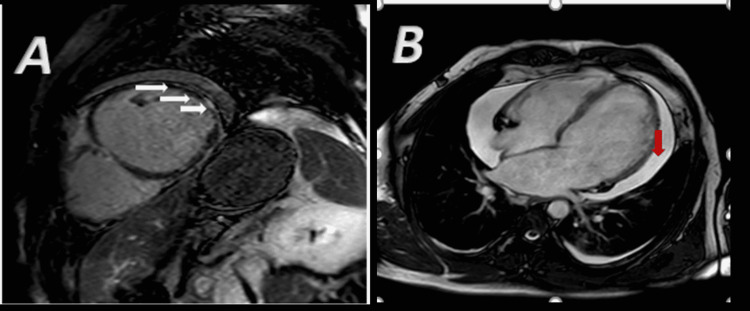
CMR demonstrates diffuse subepicardial gadolinium enhancement (white arrows) in short axis (A) and apical four-chamber view (B) compatible with myocarditis Note the presence of pericardial effusion (red arrow). CMR: cardiac magnetic resonance
